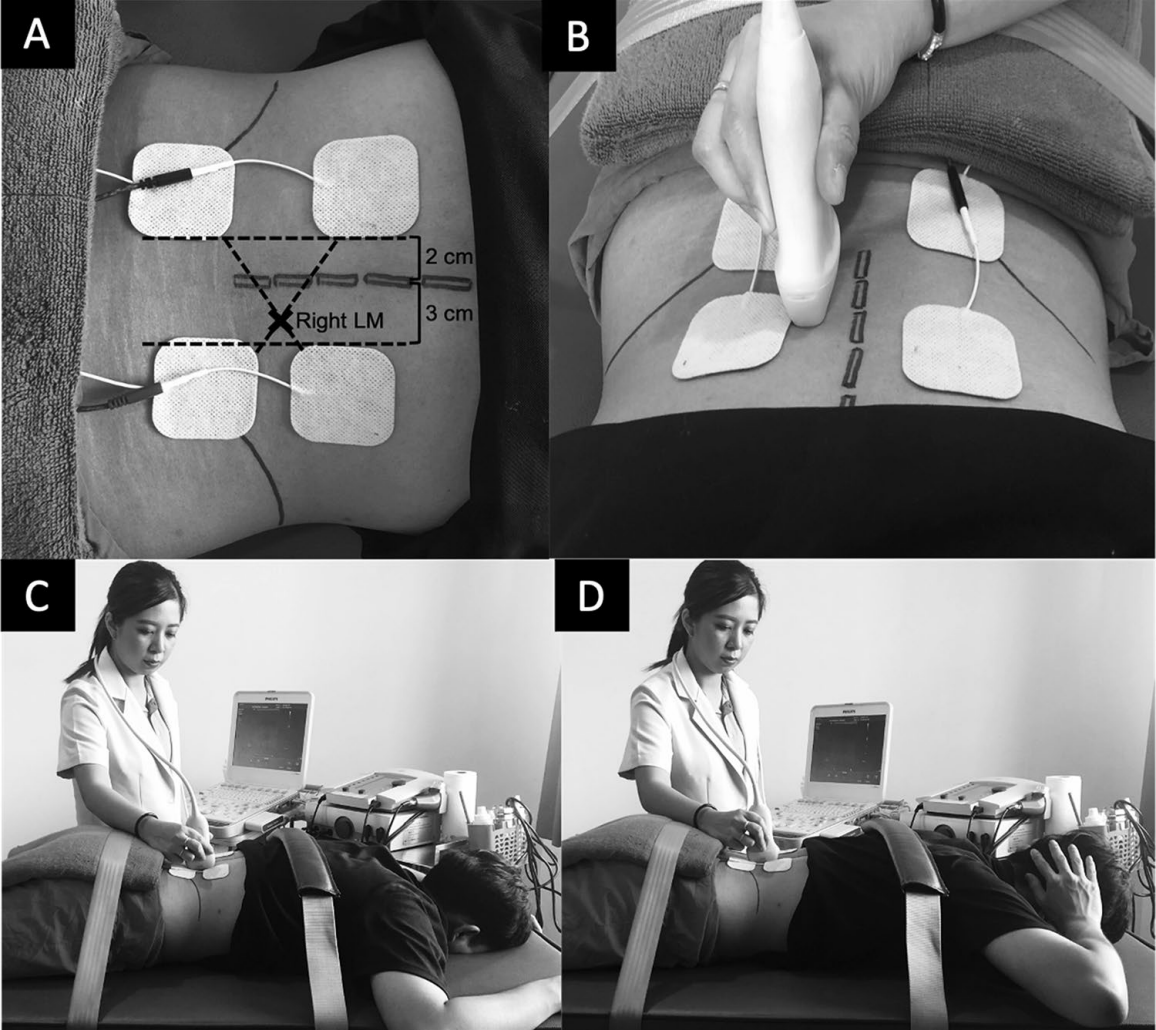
Neuromuscular electrical stimulation placement to stimulate right lumbar multifidus muscle (A) and ultrasound transducer placement at right L4-5 facet joint (B) during resting (C) and maximum voluntary isometric contraction with and without stimulation (D).
